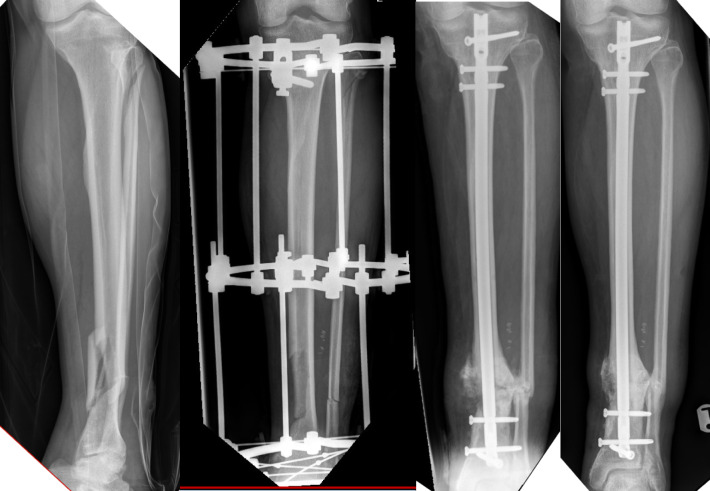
From left to right: initial injury, initial management with Ilizarov frame, acute osteotomy, biological augmentation and antibiotic coated IMN, final plain films
